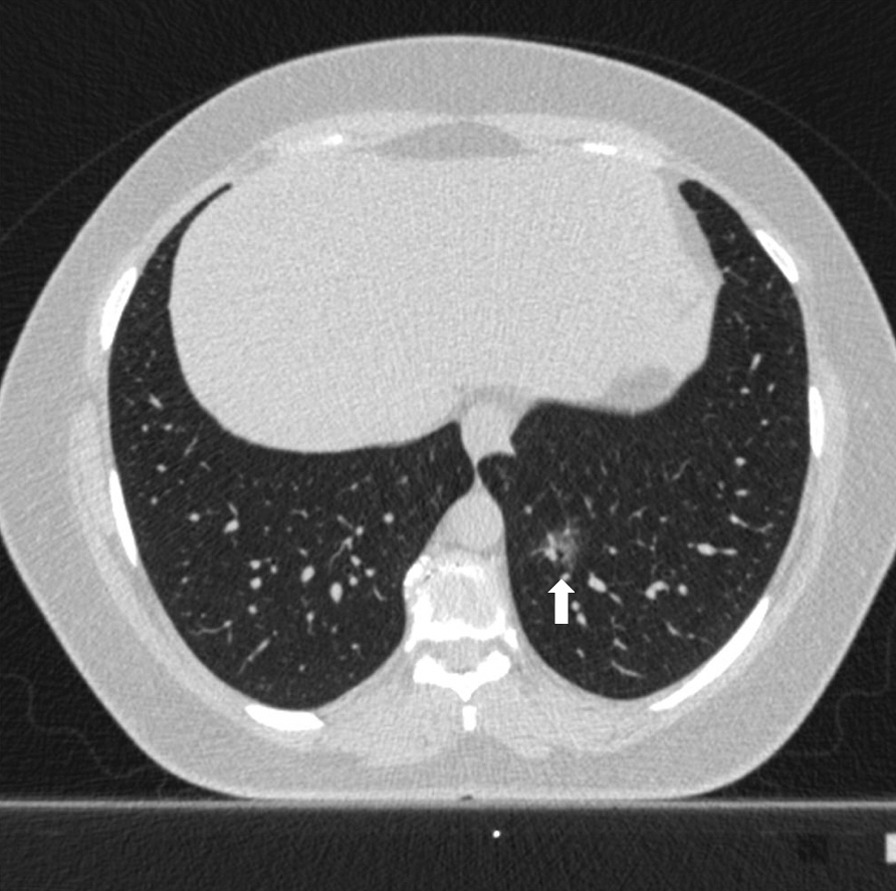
56 years old female patient. According to the CO-RADS category in terms of Covid pneumonia, a CO-RADS 3 patient had a ground glass parenchyma pattern (pattern 1) with a central lesion (white arrow), and the patient's total CT score value was 1 and the structured total CT score value was 1 according to HRCT scan
